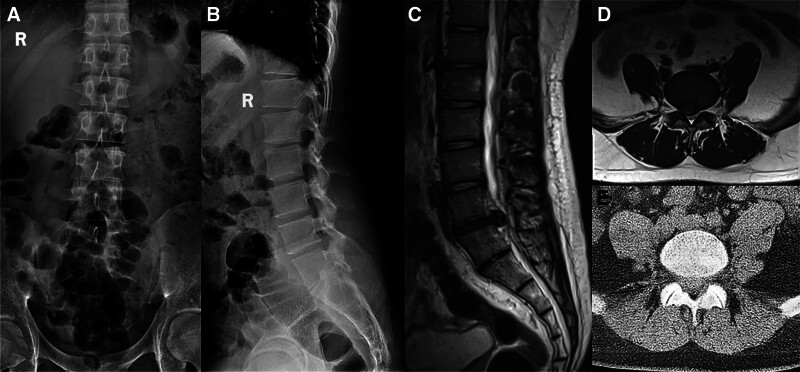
Preoperative imaging. Preoperative anteroposterior (A) and lateral (B) radiographs of the lumbar spine, showing overall spinal alignment and disc space narrowing at L5/S1. (C) Preoperative sagittal MRI T2-weighted image, demonstrating lumbar disc herniation and reduced disc height at L5/S1. Axial MRI T2-weighted image (D) and axial CT scan (E) revealing the L5/S1 disc herniation and associated nerve root compression. MRI = magnetic resonance imaging.
